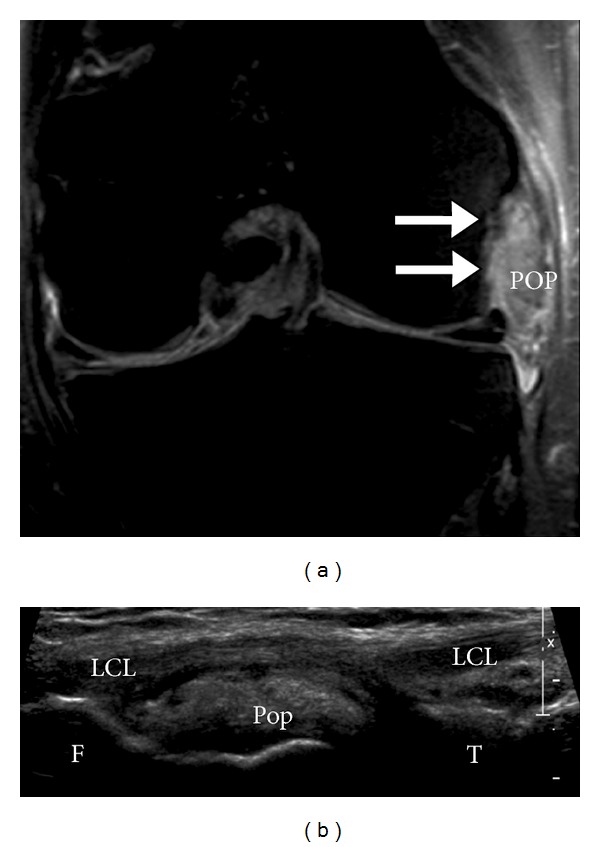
Gout involving intra-articular popliteus tendon in the knee. Coronal fat saturated T2 MR image (a) demonstrates intermediate signal gout deposit in the popliteus tendon (Pop) adjacent to the popliteus groove (arrows), deep to the lateral collateral ligament (LCL). Note the hyperechogenicity of the gout deposit on ultrasound (b). F: femur; T: tibia.
